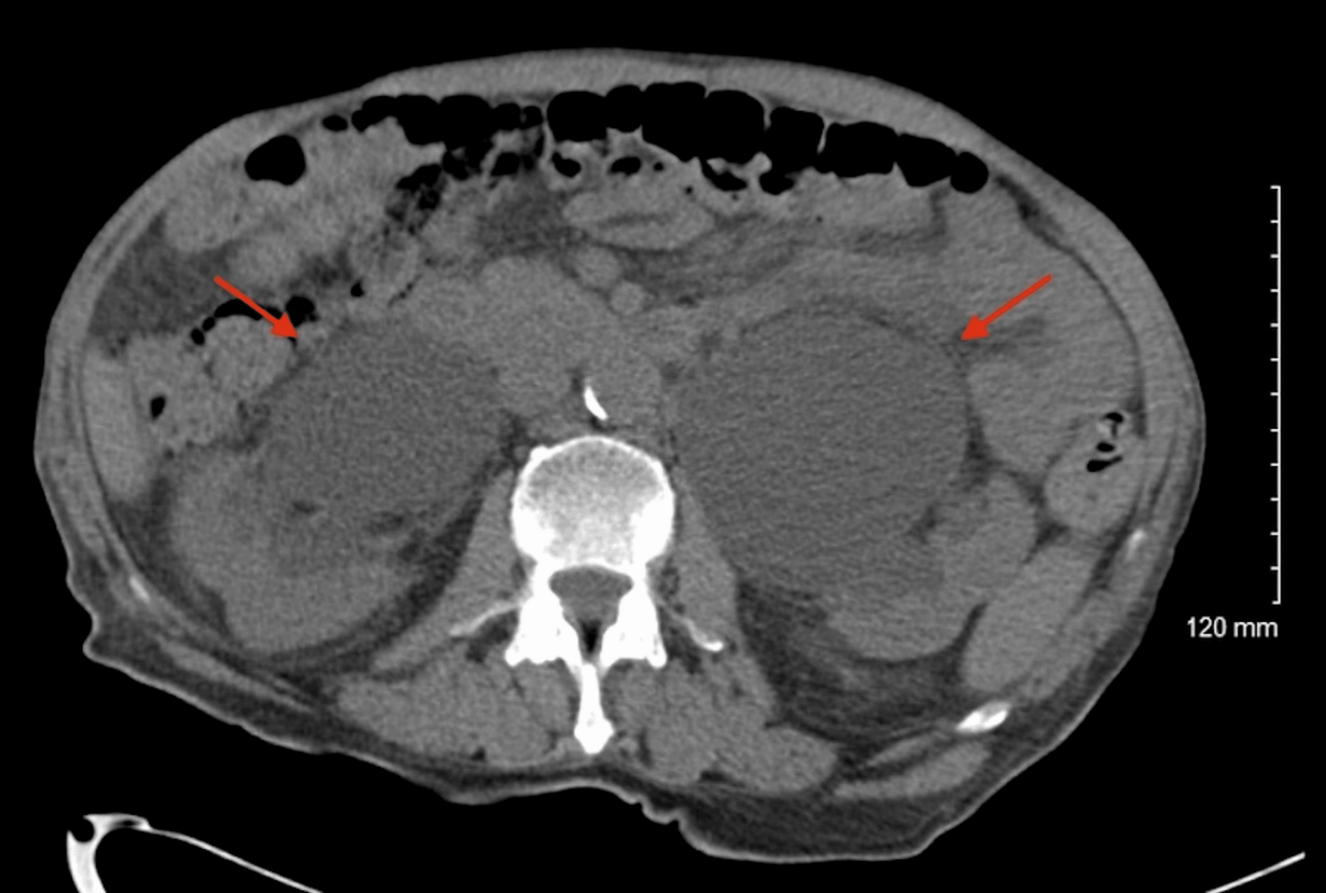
Abdominal CT image on admission revealing marked bilateral hydronephrosis and hydroureter (red arrows).
